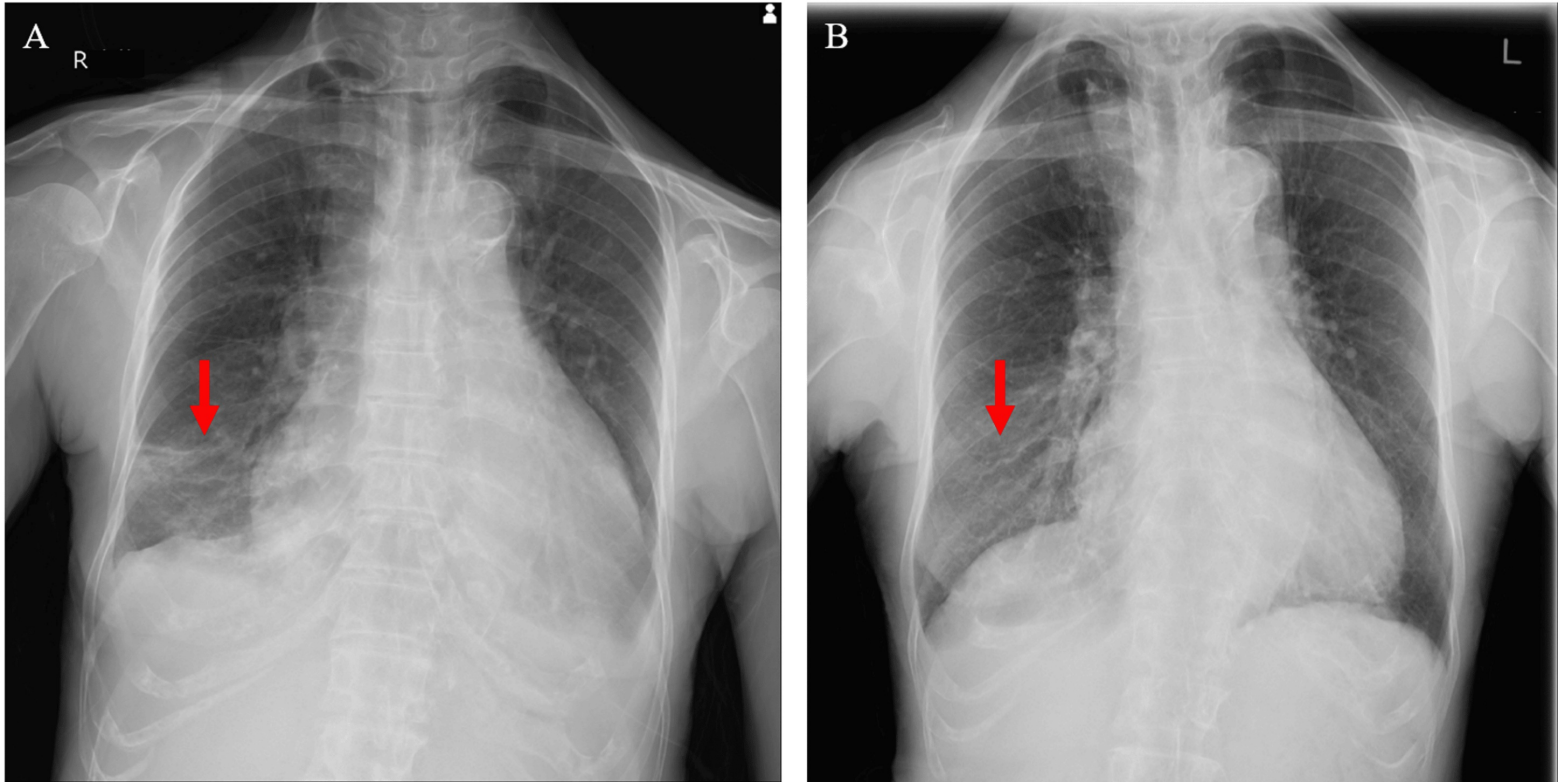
Chest X-ray after pleural drainage A: X-ray image immediately after pleural drainage. B: X-ray image taken four months later.
